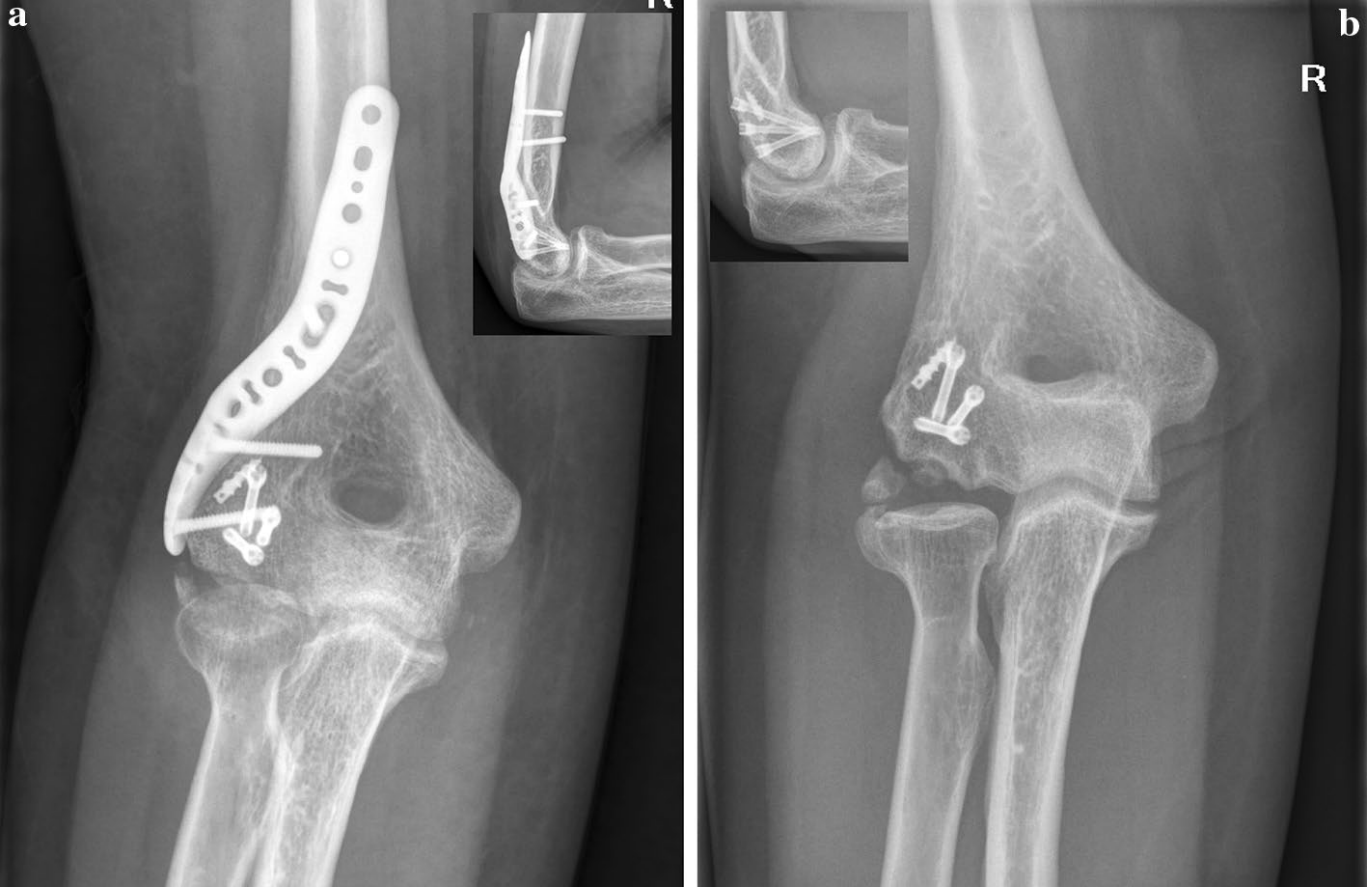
Case of a 33-year old female patient who developed partial avascular necrosis. A Plain radiographs 3 months postoperatively. B Plain radiographs following partial implant removal and arthrolysis
